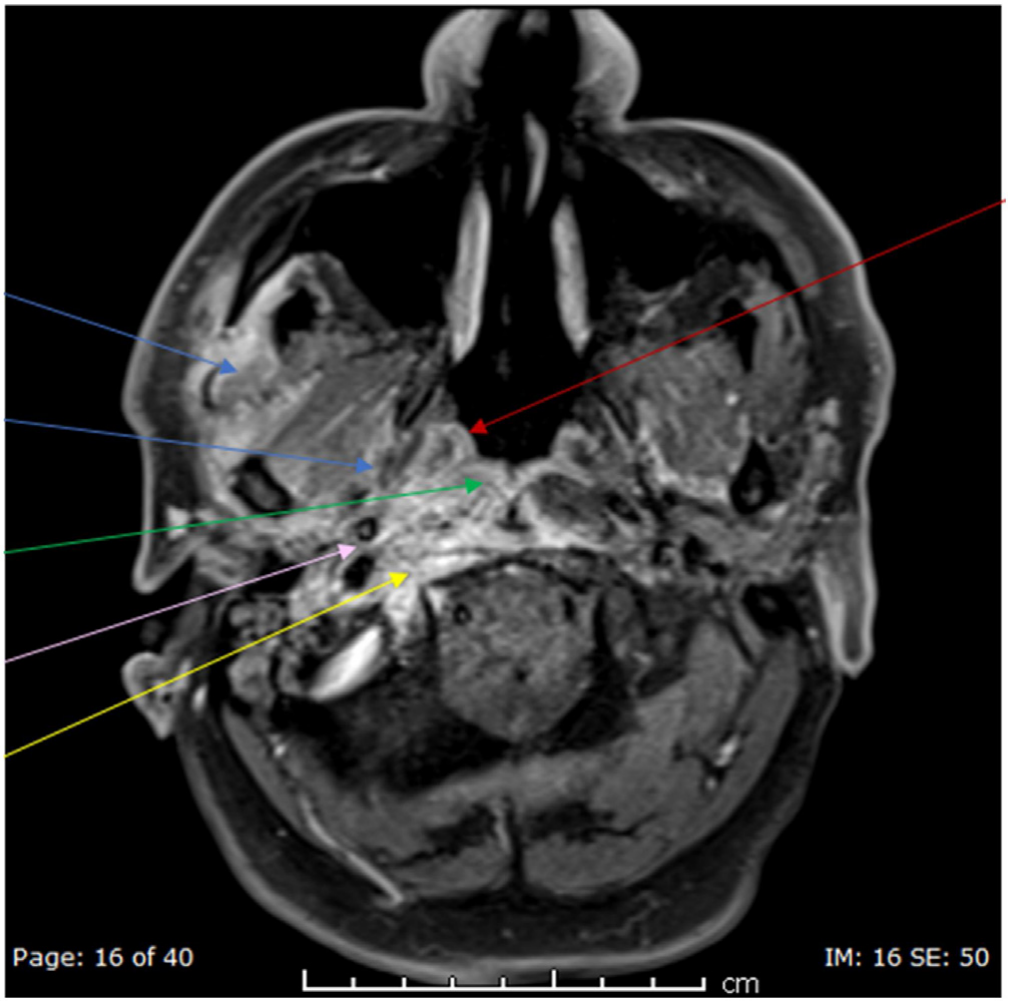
Axial and coronal post contrast enhanced MRI scan confirming diffuse multicompartmental involvement with enhancement in the masticator and parapharyngeal spaces as described previously (blue arrows). In addition, there is better delineation of medial and posterior extension including pharyngeal mucosal space of the nasopharynx (red arrow), retropharyngeal space (green arrow) and perivertebral space (yellow arrow). Note the enhanacment in the right carotid space surrounding IJV and ICA (pink arrow). Coronal image delineates extension into right TMJ (purple arrow) explaining erosion or upper condylar head margin. Note no evidence of meningeal enhancement (orange) therefore no convincing intracranial extension. The diffuse nature of disease raises the possibility of an infective cause rather than neoplastic. (For interpretation of the references to colour in this Figure legend, the reader is referred to the web version of this article).
